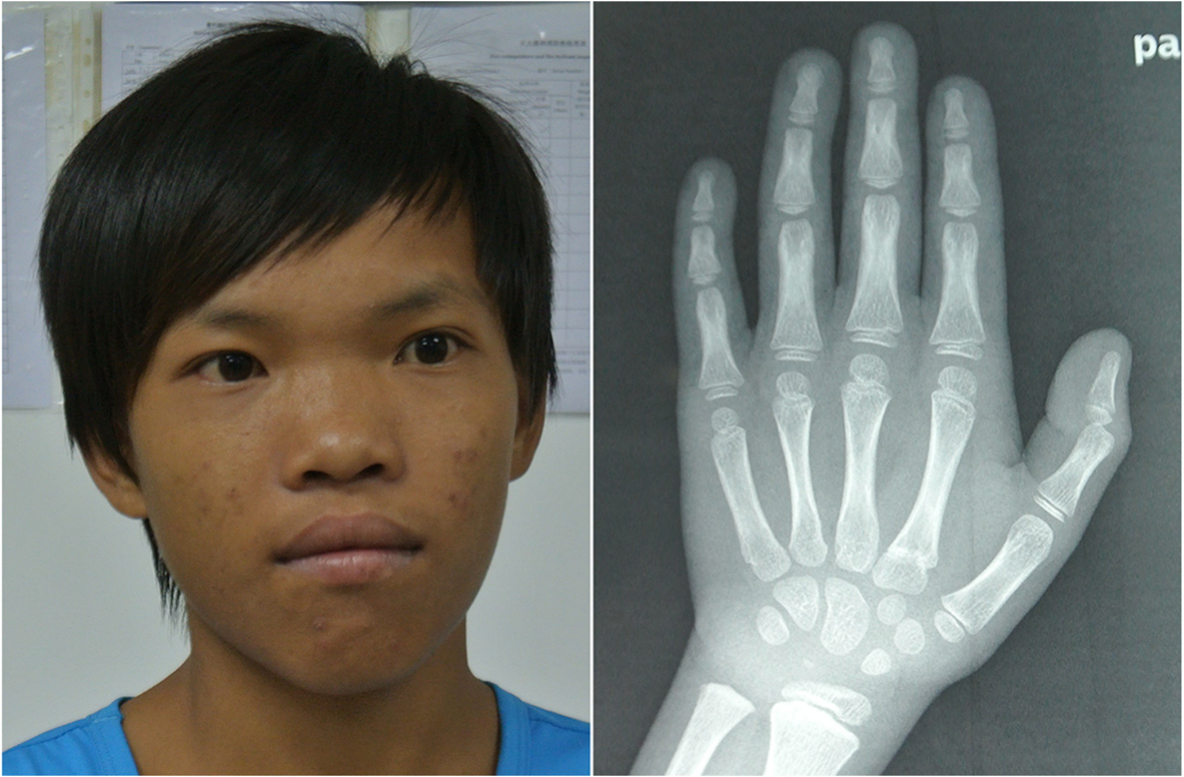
The probandat 14 years of age. Note relative macrocephaly, coarse face, broad forehead, deep-set and widely spaced eyes, strabismus, broad nose bridge, shallow philtrum, thick lips. Hand X-ray showed delayed bone age.
